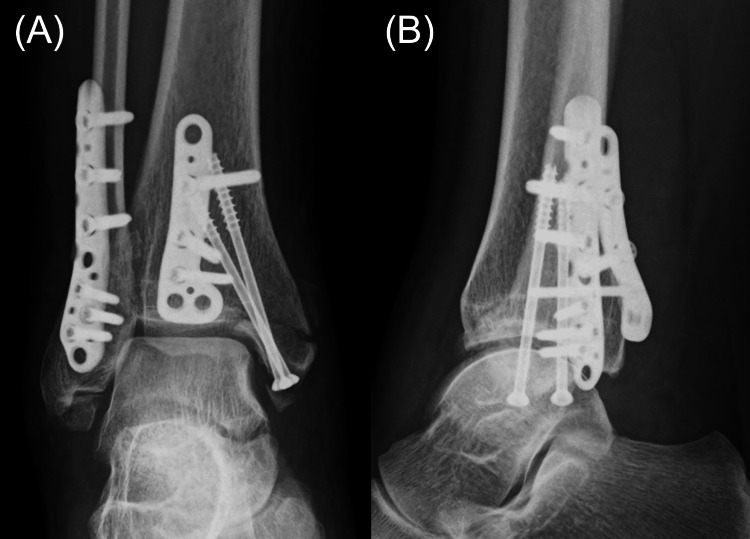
Plain radiographs of the right ankle in (A) anteroposterior and (B) lateral views immediately after fracture surgery The posterior and lateral malleolar fragments can be seen fixed with a plate and the medial malleolar fragment is seen fixed with screws.
